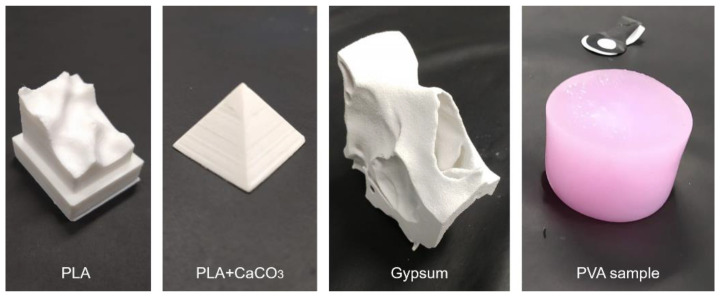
Pictures of the X-ray sample materials that have been tested.
